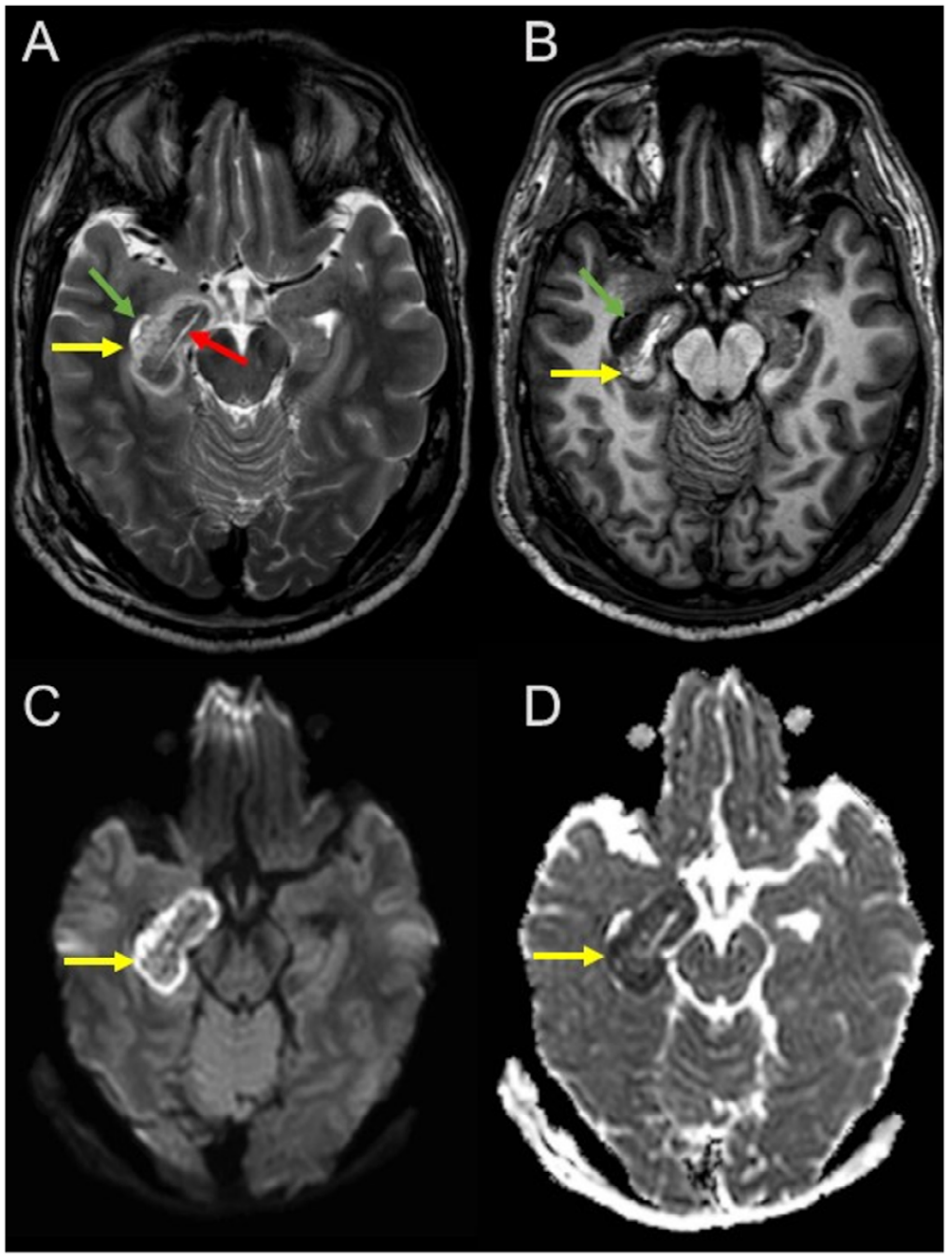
Introduction example. Early postoperative MRI following laser interstitial thermal therapy in a patient with right‐sided temporal lobe epilepsy. In the right amygdala and hippocampal region beside the temporal horn (green arrow), a central elongated early postoperative defect is visible following laser ablation (red arrow) surrounded by thermal edema (yellow arrows) that closely resembles infarction‐related edema due to diffusion restriction. (A) Transversal T2‐weighted Turbo‐Spin‐Echo; (B) transversal T1‐weighted Turbo‐Field‐Echo; bottom: transversal diffusion‐weighted imaging; (C) B1000; (D) apparent diffusion coefficient map.
